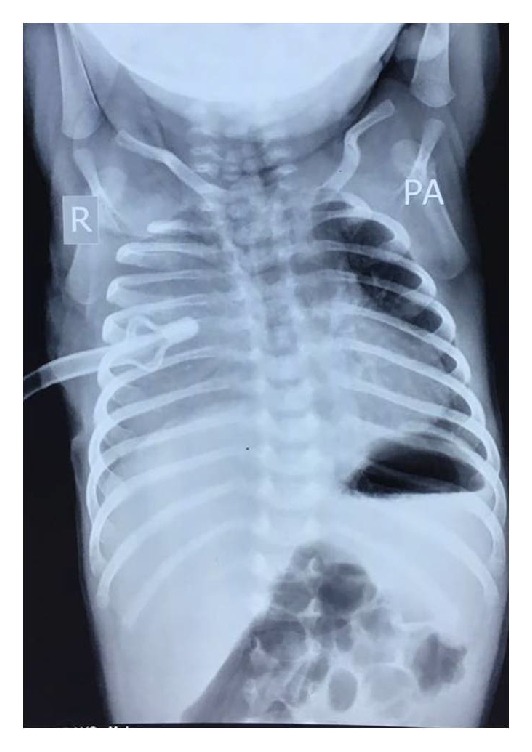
Chest radiograph showing a homogeneous opacity in the right middle and lower lung fields with shift of mediastinum to left.
